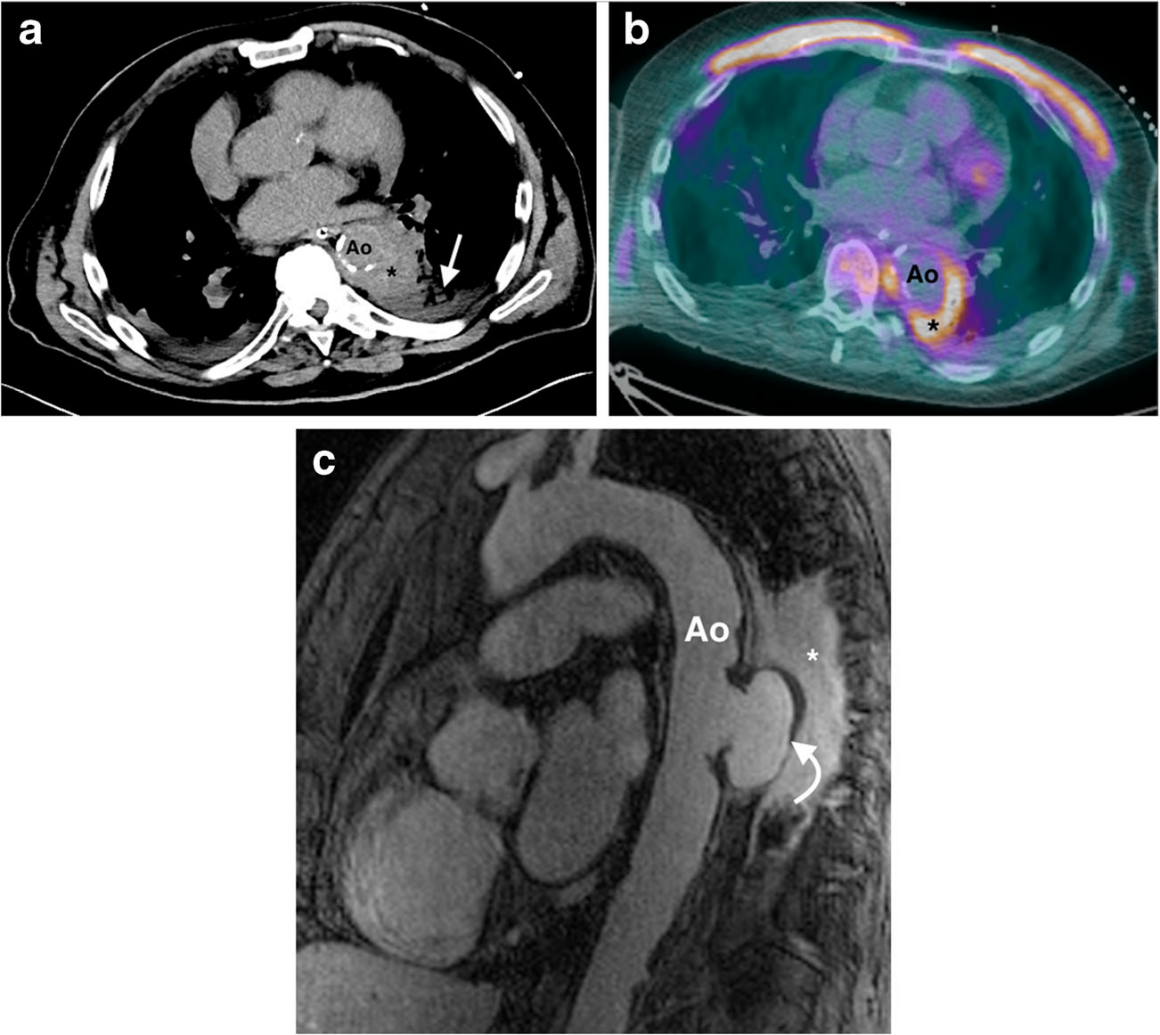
A 78-year-old man presents with chest pain, fever and *S. aureus* septicemia. (a) An axial non-contrast CT thorax image demonstrates high attenuation in the descending thoracic aorta wall (*), with adjacent consolidation in the left lower lobe (*arrow*). (b) An 18 F-FDG PET/CT image shows corresponding increased FDG uptake in the aortic wall (*). The patient was diagnosed with an infected intramural haematoma, and was treated with antibiotics. (c) A follow-up sagittal fat-saturated T1 MRI post-GBCA image shows development of a large saccular pseudoaneurysm (*curved arrow*), with persistent adjacent inflammatory tissue (*). The patient subsequently underwent surgical repair. *Ao = descending thoracic aorta*
